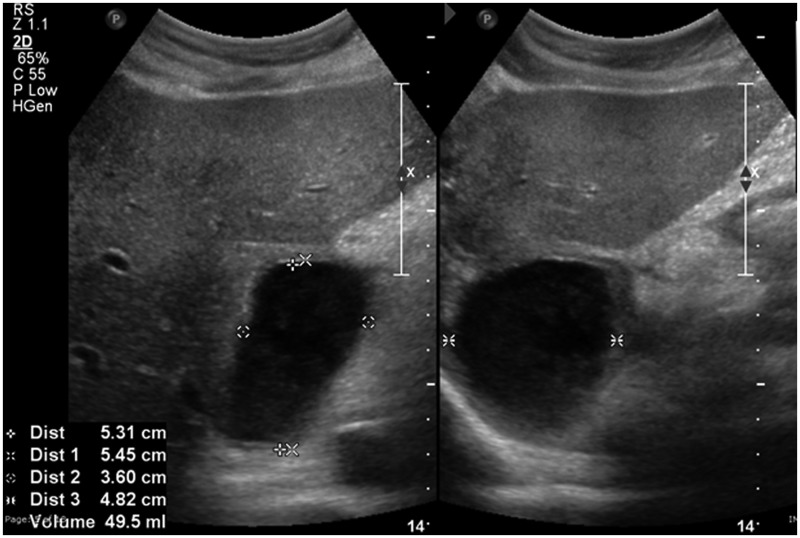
Ultrasound image showing pseudocyst in the caudate lobe
